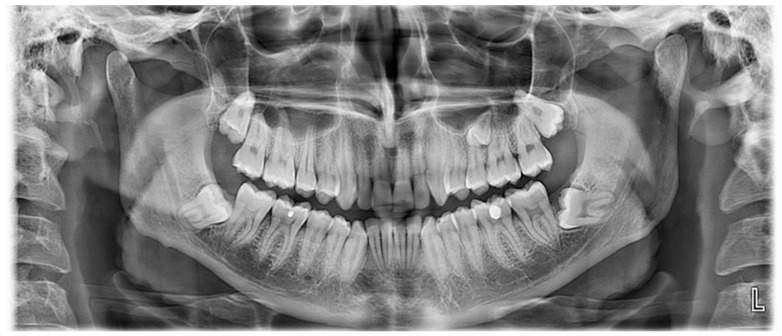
The four impacted third molars and the impacted paramolar are seen in the panoramic radiograph. The root of the paramolar seems to be close to the sinus maxillary wall.
